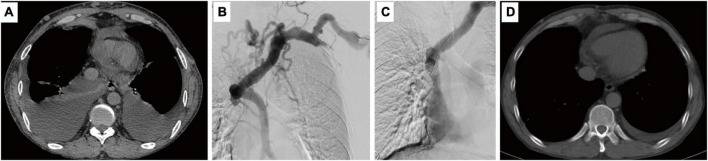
Computed tomography shows pleural effusion (A) and angiography shows superior vena cava occlusion (B). Balloon dilatation was performed (C) and pleural effusion no longer appeared after 4 months (D).
